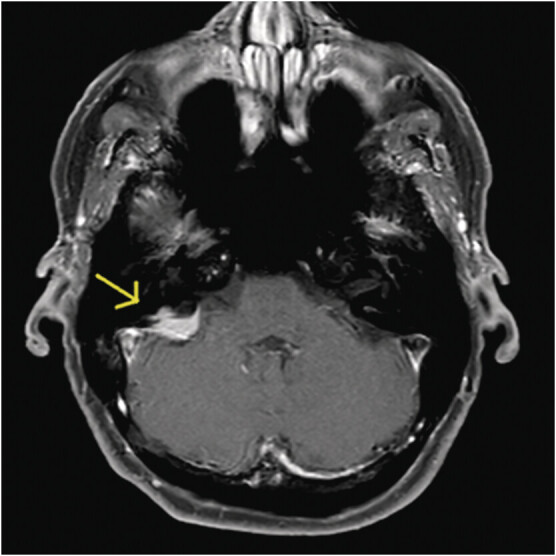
T1 postcontrast MRI image showing meningioma with invasion of the vestibular aqueduct.
